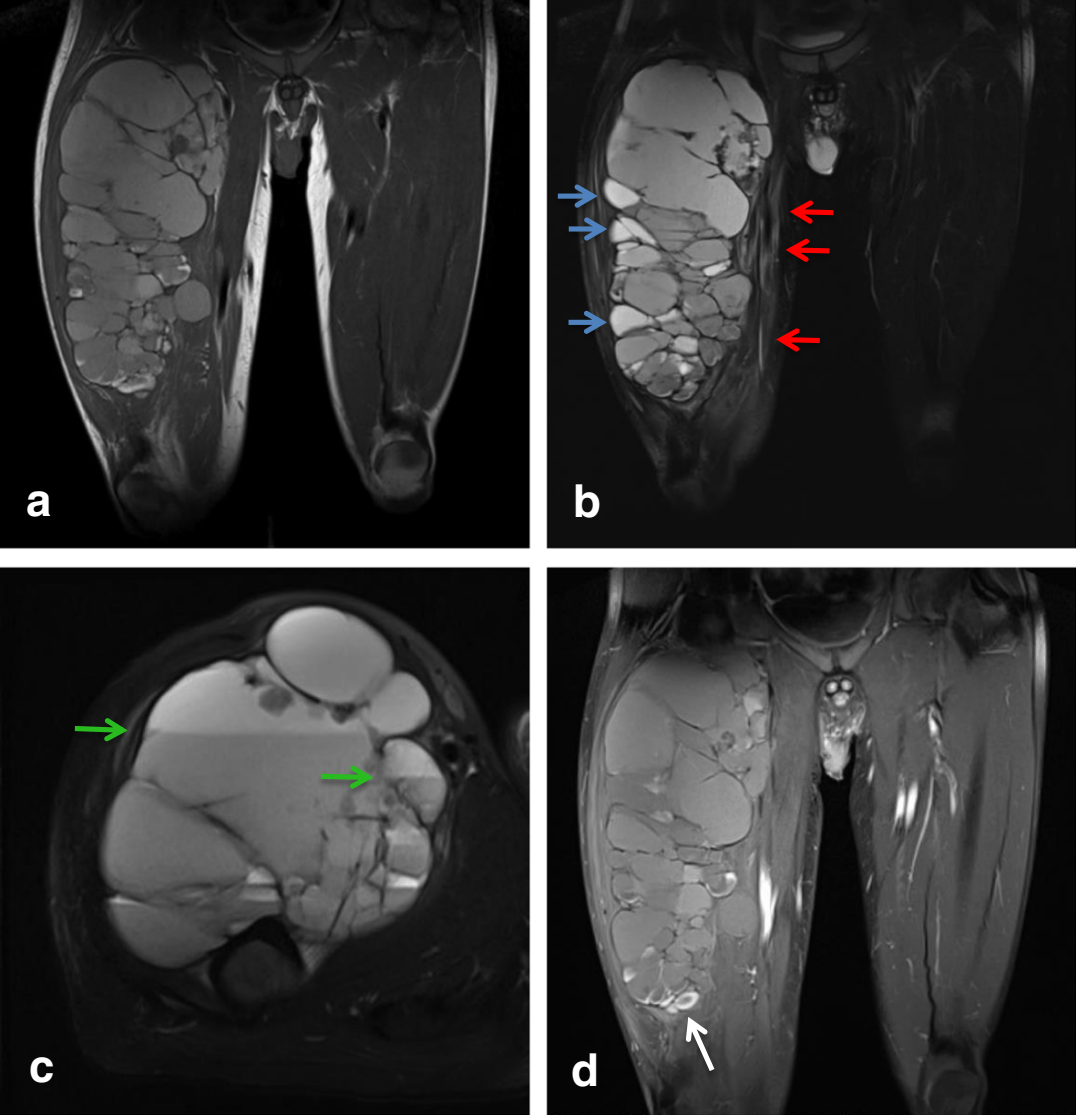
A 34-year-old man diagnosed with angiomatoid fibrous histiocytoma (case 6): (a) T1-weighted spin echo, (b, c) T2-weighted spin echo, and (d) contrast-enhanced images. A 163 × 130 × 303-mm painless mass is present in the vastus intermedius of the right thigh. The lesion is heterogeneously iso-hyperintense on T1 WI and presents with a multilocular area, cystic area (*blue arrows*), fluid–fluid level (green arrows), pseudocapsule, and peritumoral edema (*red arrows*) on T2 WI. A contrast-enhanced image shows partial gadolinium enhancement (*white arrows*)
